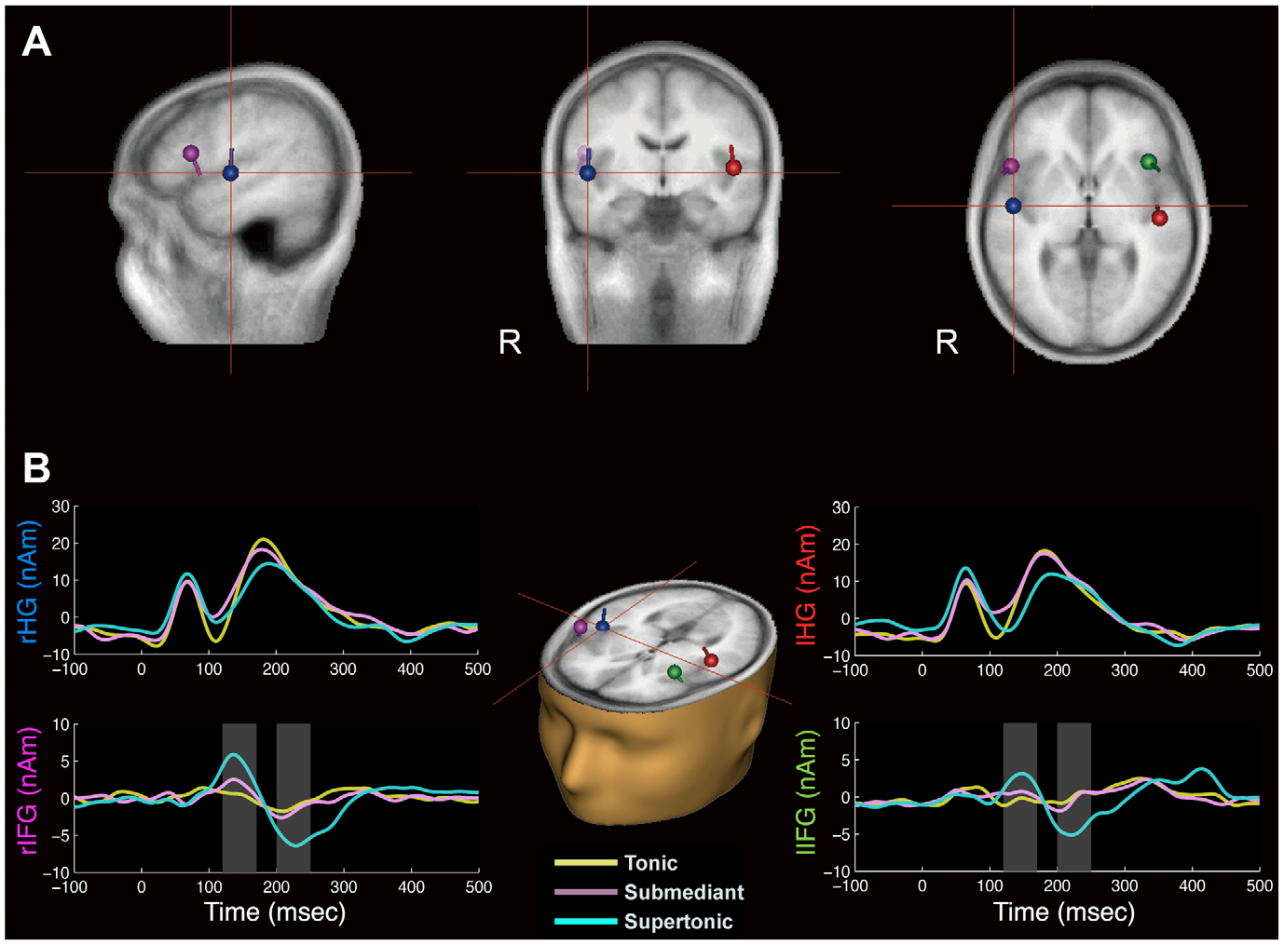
Locations and activities of multiple ECDs. A. Multiple ECDs of an individual superimposed on a sagittal (left panel), a coronal (middle panel) and an axial (right panel) plane of stereotactic template MRI orientated in a radiological convention (left to right). ECDs are located on the left Heschl's gyrus (red), the right Heschl's gyrus (blue), the left inferior frontal gyrus (green) and the right inferior frontal gyrus (magenta). B. Averaged ECD activities across all participants (n = 19) for tonic (yellow), submediant (purple) and supertonic (cyan) conditions. The shaded areas indicate the time-windows used in statistical analysis.
